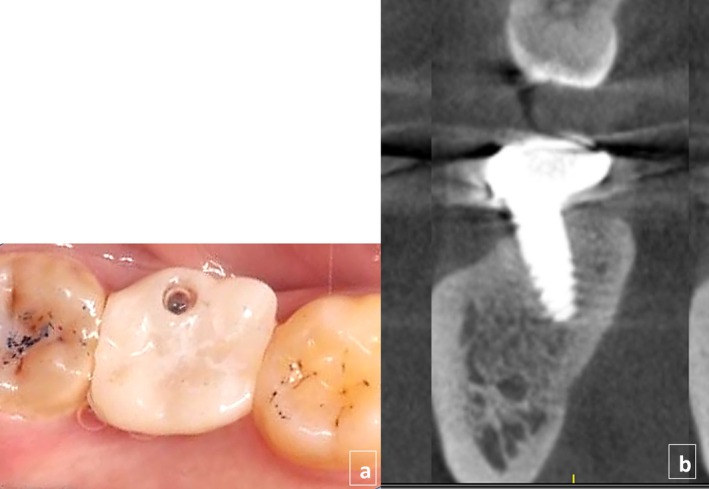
(a) Final zirconia crown; (b) CBCT coronal view of the bone healing after 2 years.
